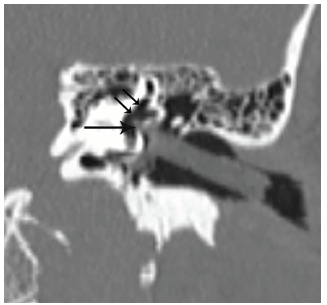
Temporal bone computed tomography reconstructed in an oblique coronal view shows a linear foreign body projecting from the external auditory canal to the oval window, with a small projection into the vestibule (arrow). Extensive intralabyrinthine air is present (double arrows).
